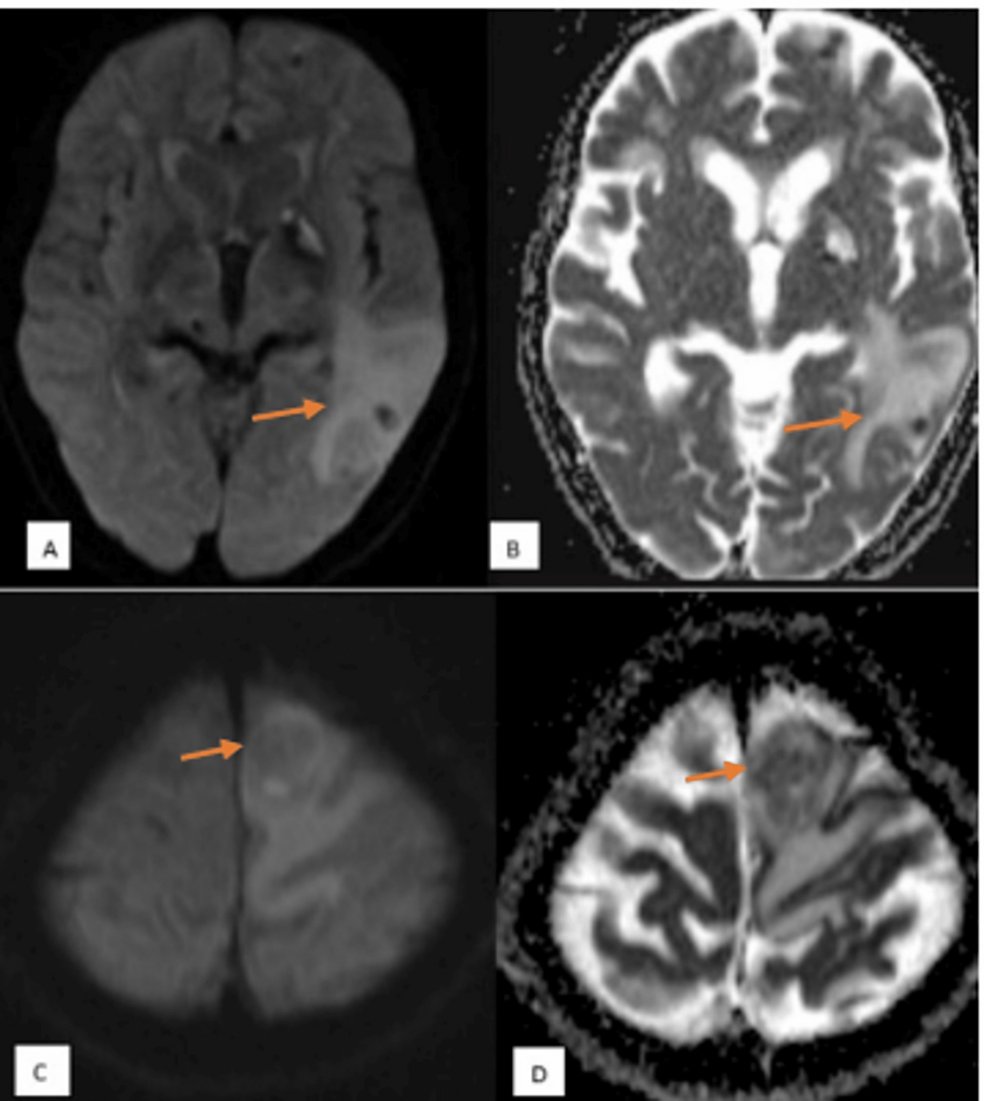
Diffusion-weighted imaging features and corresponding apparent diffusion coefficient (ADC) values in neurocysticercosis and tuberculoma MRI of the brain axial sections - (A) axial diffusion-weighted imaging (DWI) and (B) axial ADC map showing no restriction of diffusion (orange arrows in A and B). ADC value obtained was 1.3 x 10^-3^ mm^2^/s - case of neurocysticercosis. MRI of the brain axial sections - (C) axial DWI and (D) axial ADC map showing patchy areas of restriction of diffusion (orange arrows in C and D). ADC value obtained was 0.73 x 10^-3^ mm^2^/s - case of tuberculoma.
